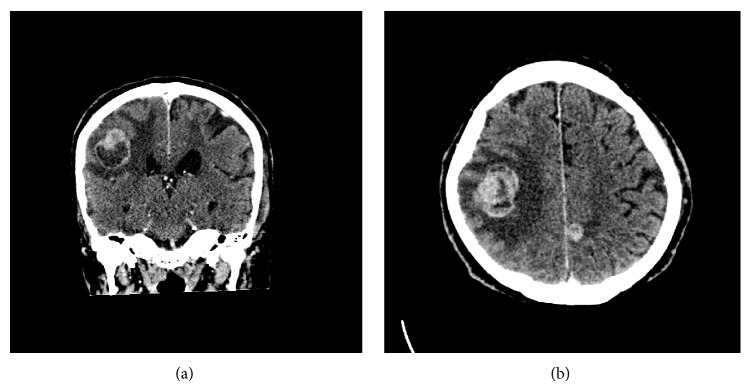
CT imaging of brain metastases in the coronal (a) and axial (b) planes.
